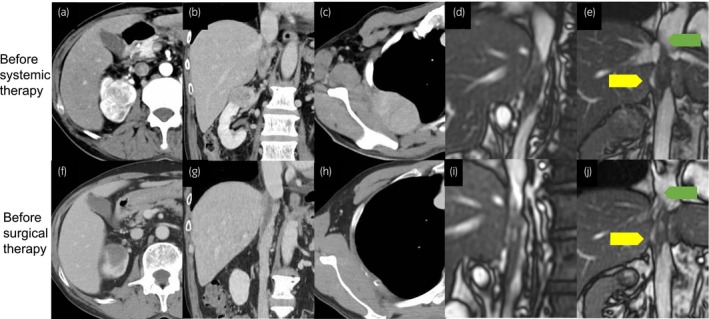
Imaging manifestations of renal cancer, the IVC thrombus, and the fourth rib metastases. (a–c) Computed tomography before systemic therapy. (d, e) MRI before surgery. (f–h) Computed tomography before systemic therapy. (i, j) MRI before surgery. The yellow arrows denote the blood flow between the IVC wall and thrombus, and the green arrows denote the absence of blood flow between the wall and the advanced portion.
